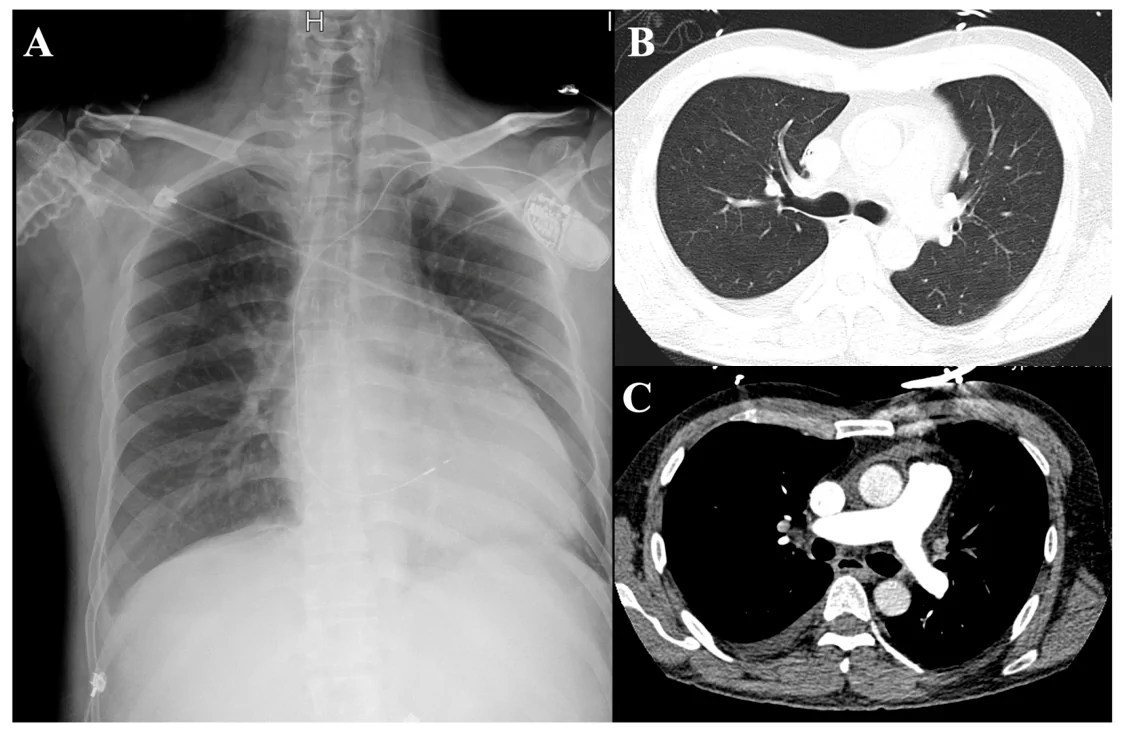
Imaging evaluation of hypoxemia etiology. (A) Chest radiograph showing no acute pulmonary edema. (B) Chest CT showing no significant pulmonary infection. (C) CT pulmonary angiography excluding pulmonary embolism.
